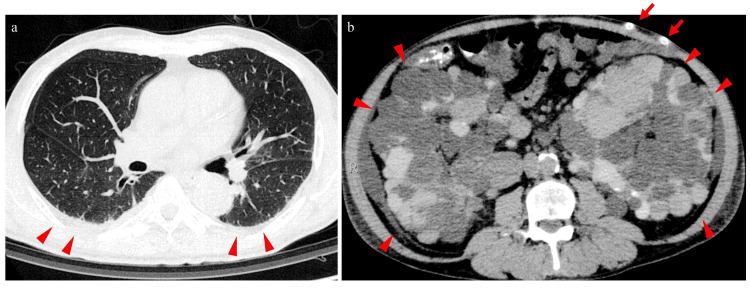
Computed tomography findings at admission (a) Chest computed tomography showed no notable abnormalities except for mild pleural effusion. (b) Abdominal computed tomography showed no notable abnormalities except for the presence of a peritoneal dialysis catheter (arrows) and numerous renal cysts (arrowheads).
